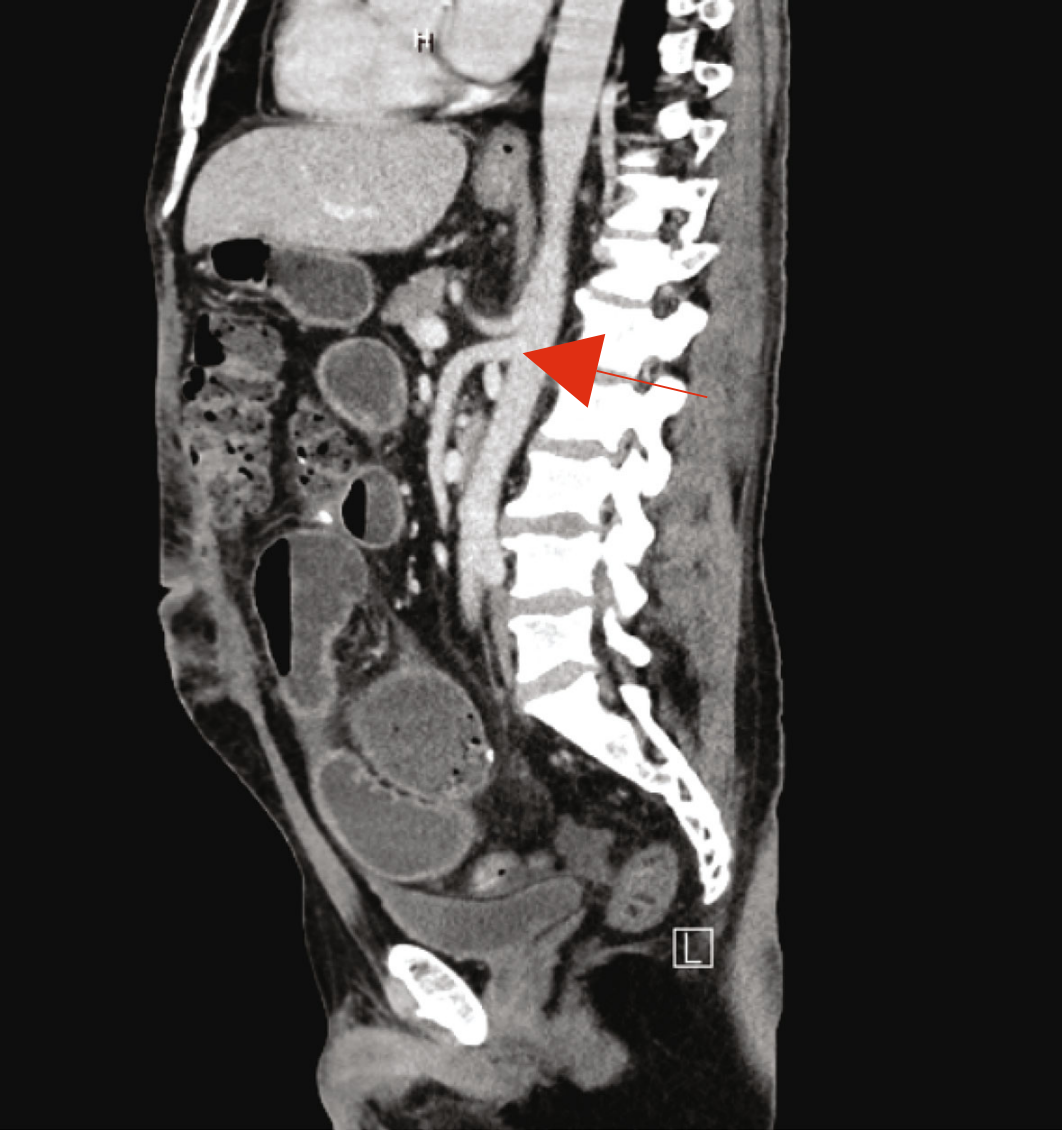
Contrast-enhanced CT demonstrating patent superior mesenteric and celiac arteries.
